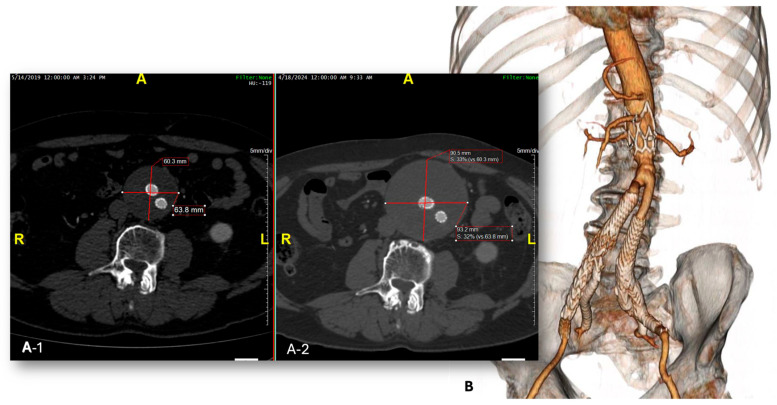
Control CTA showing evident enlargement of the aneurysmal sac due to type II endoleak (A1,A2). Partial surgical conversion with explantation of the endograft main body and preservation of the iliac side branches at 103 months after the index procedure (B).
